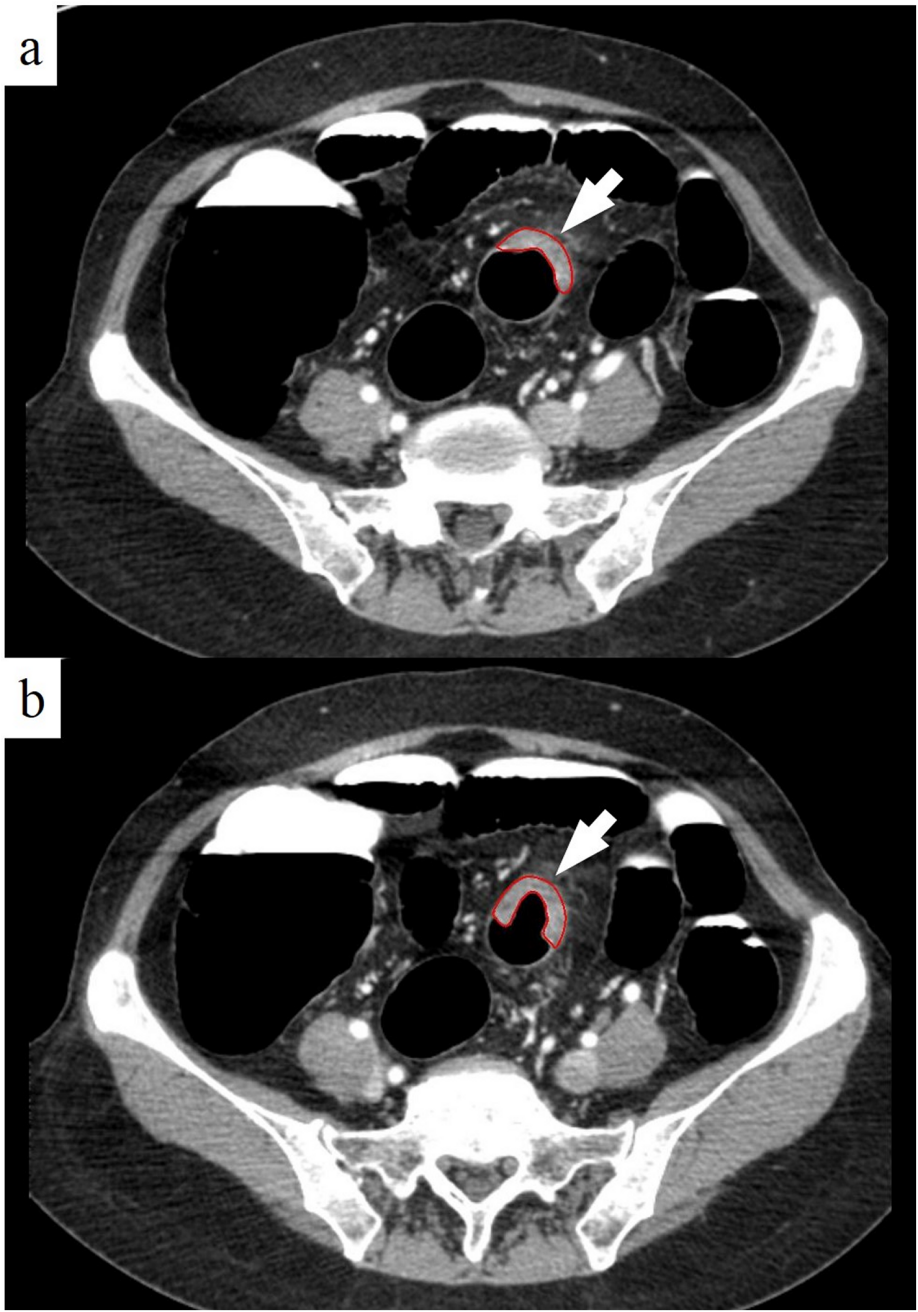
Volume measurement using CT colonography. a, b. Eccentric enhancing wall thickening (arrow) of the sigmoid colon is well demonstrated on axial CT images in prone position. Regions of interest (ROIs) (red line) are drawn covering abnormal wall thickening on every contiguous CT slices. Volume (cm^3^) is calculated by multiplying areas (cm^2^) and reconstruction interval (cm).
